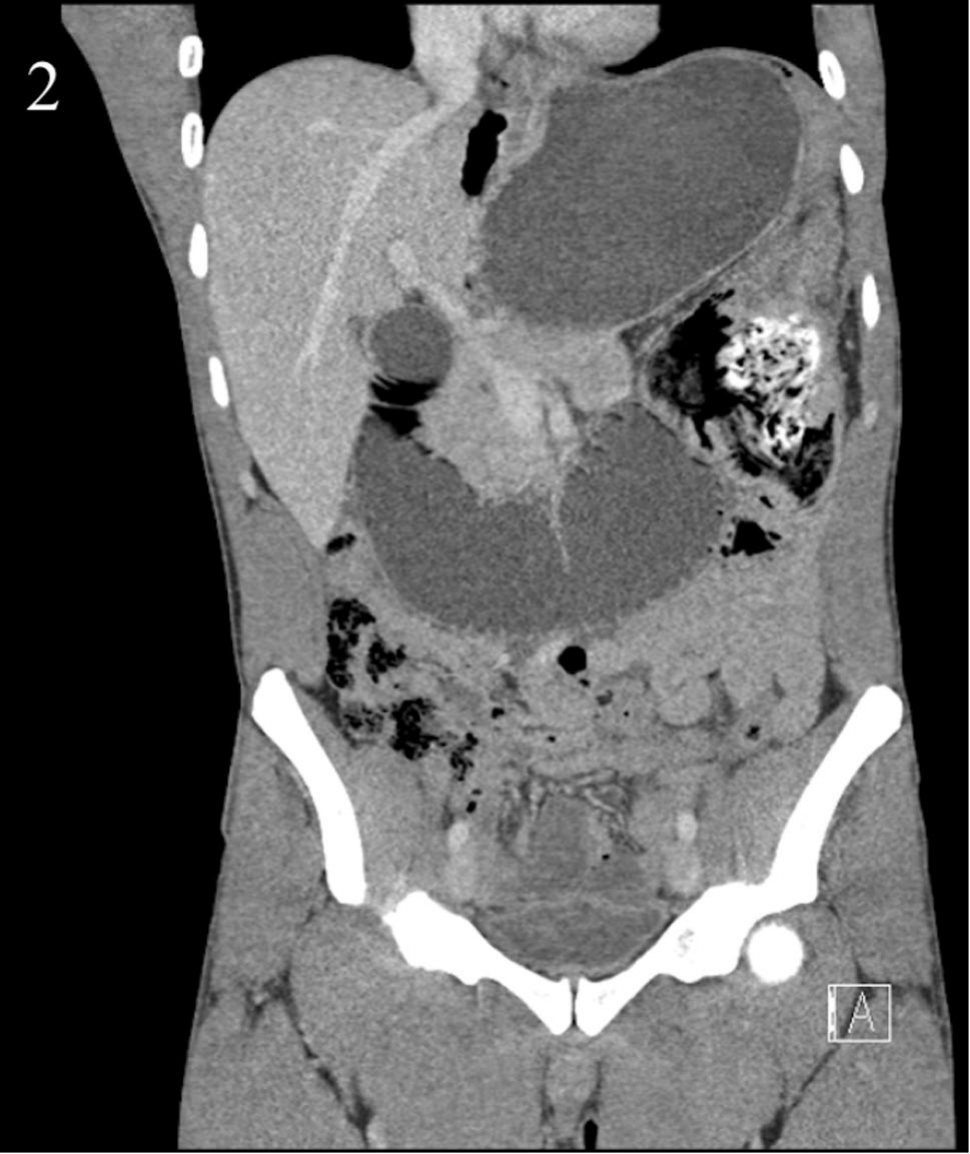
Coronal CT image showing proximal duodenal and gastric distension secondary to heterogenous intraluminal mass at the duodenal jejunal flexure/proximal jejunum which is suspicious for gossypiboma
